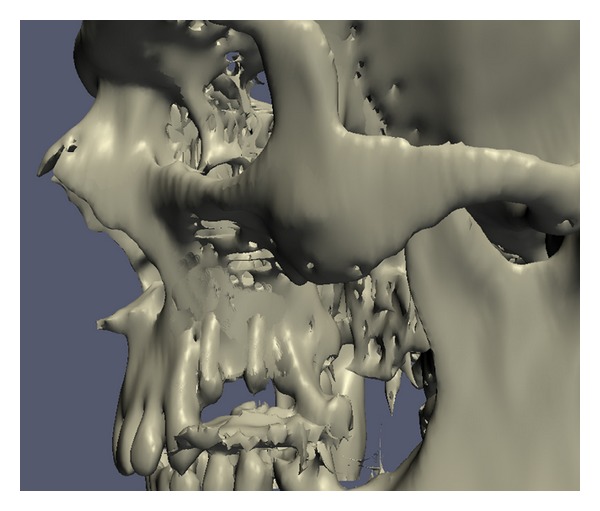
CT scan view after implant placement.
